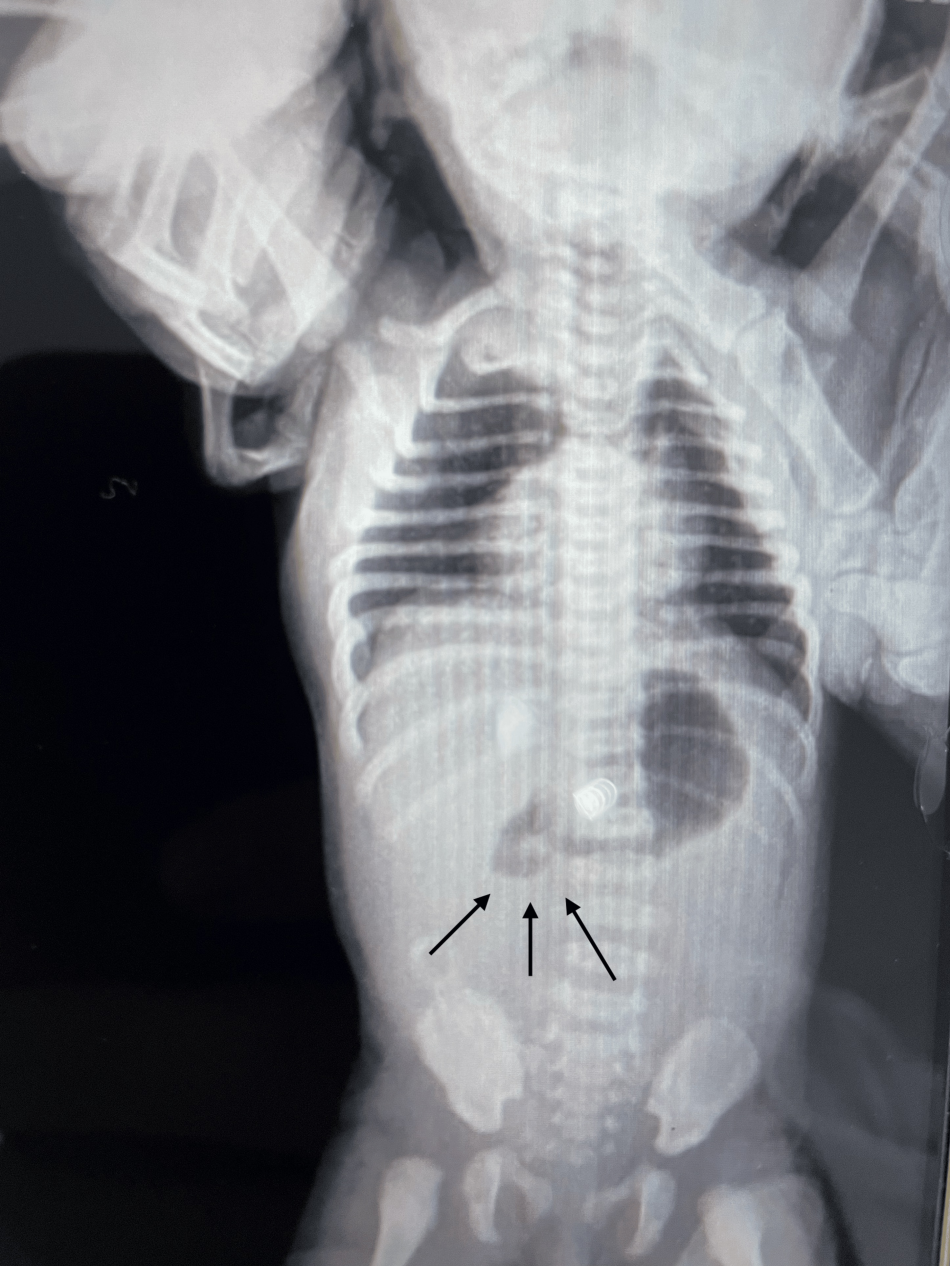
Abdominal X-ray showing absence of distal bowel gas (arrows), suggestive of intestinal obstruction.
